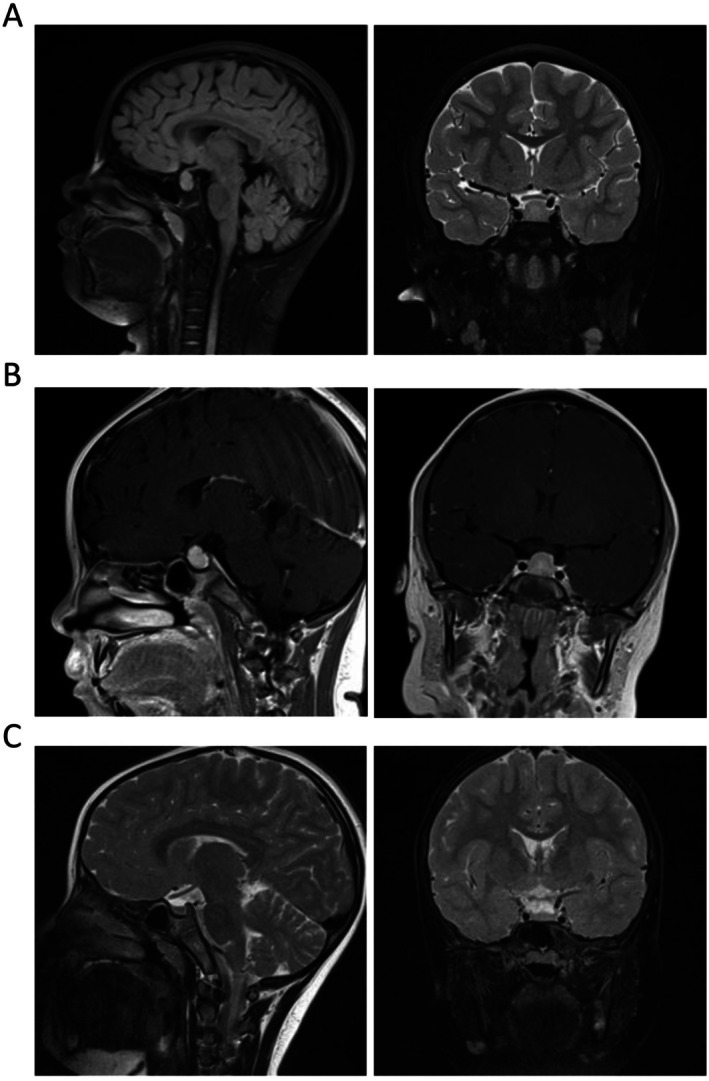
Brain magnetic resonance imaging (MRI) at diagnosis and after 3 months of levothyroxine replacement. (A) Pituitary hyperplasia at diagnosis (craniocaudal diameter: 13.5 mm, slightly compressing the optic chiasm). (B) Enhanced MRI with pituitary slices excluded gigantism and pituitary adenoma as a cause for early puberty. (C) Normal pituitary size after 3 months of levothyroxine replacement (craniocaudal diameter: 5.2 mm).
